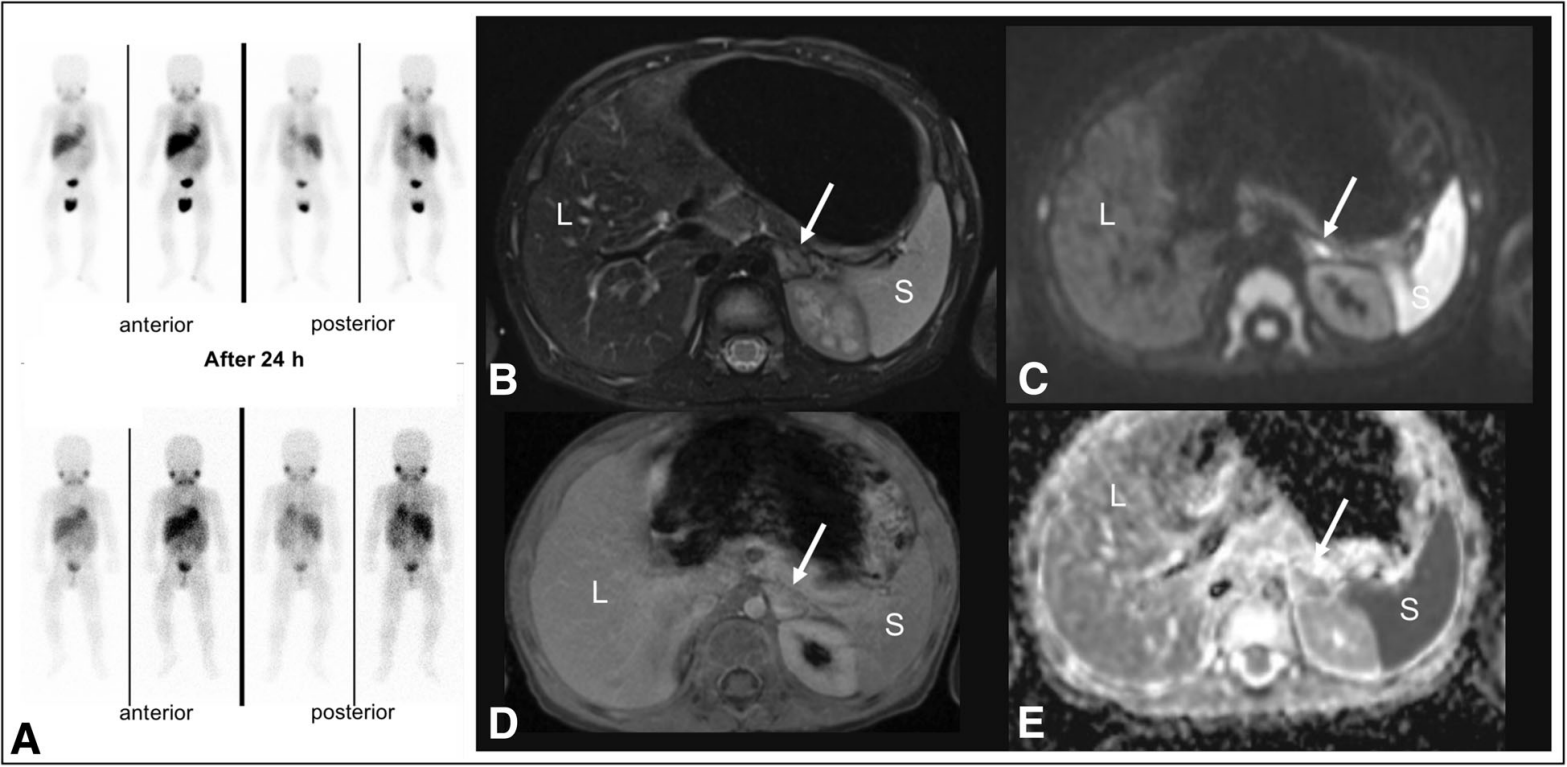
Case 2: 13 months old boy presenting with opsoclonus-myoclonus syndrome. No pathological tracer uptake could be detected in the I-123-mIBG scintigraphy with 40 mBq I-123-mIBG (a). WB-MRI revealed a T2 hyperintense mass (*white arrow*) of the left adrenal gland (b) with diffuse enhancement in the post-contrast T1 weighted sequences after the administration of 2 ml gadolinium compound (d). Restricted diffusion in diffusion imaging (c) with partial low ADC (e) could be detected. *L = liver, S = spleen*
